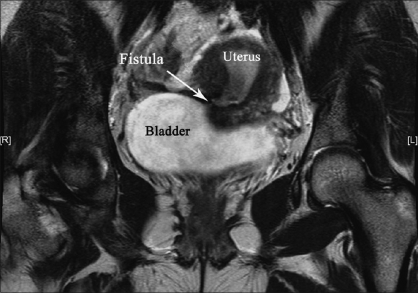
Coronal T2-weighted HR/SENSE MR image depicting the abnormal hypointense out pouching and fistulous tract (*arrow*) from posterior wall of bladder to anterolateral wall of uterus above the isthmus. Endometrial cavity is being opacified (hyperintense) with urine
